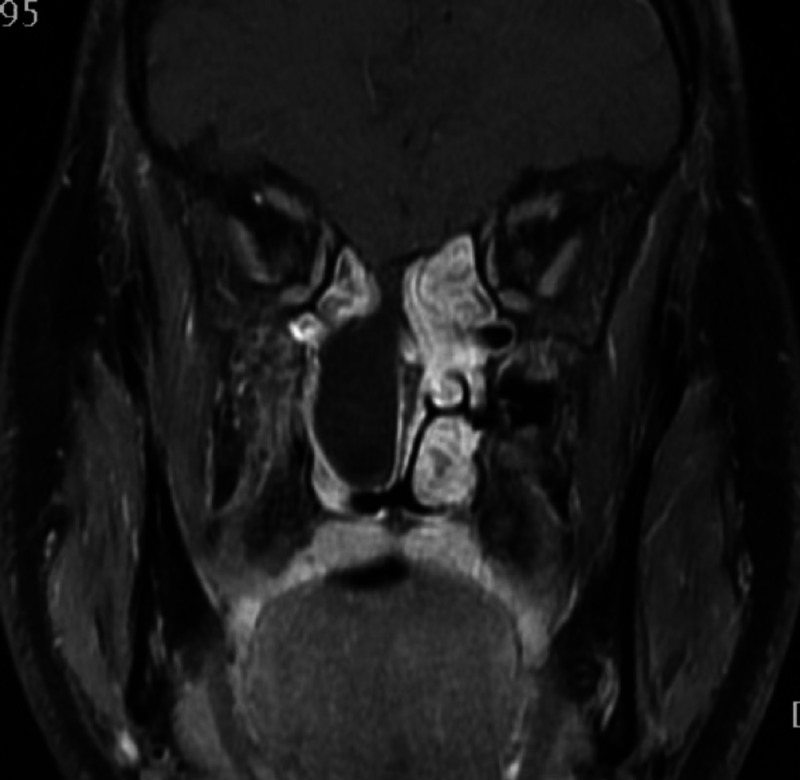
Coronal MRI sinuses confirming the presence of an ethmoid meningocele protruding through the cribriform plate defect. MRI: magnetic resonance imaging.
